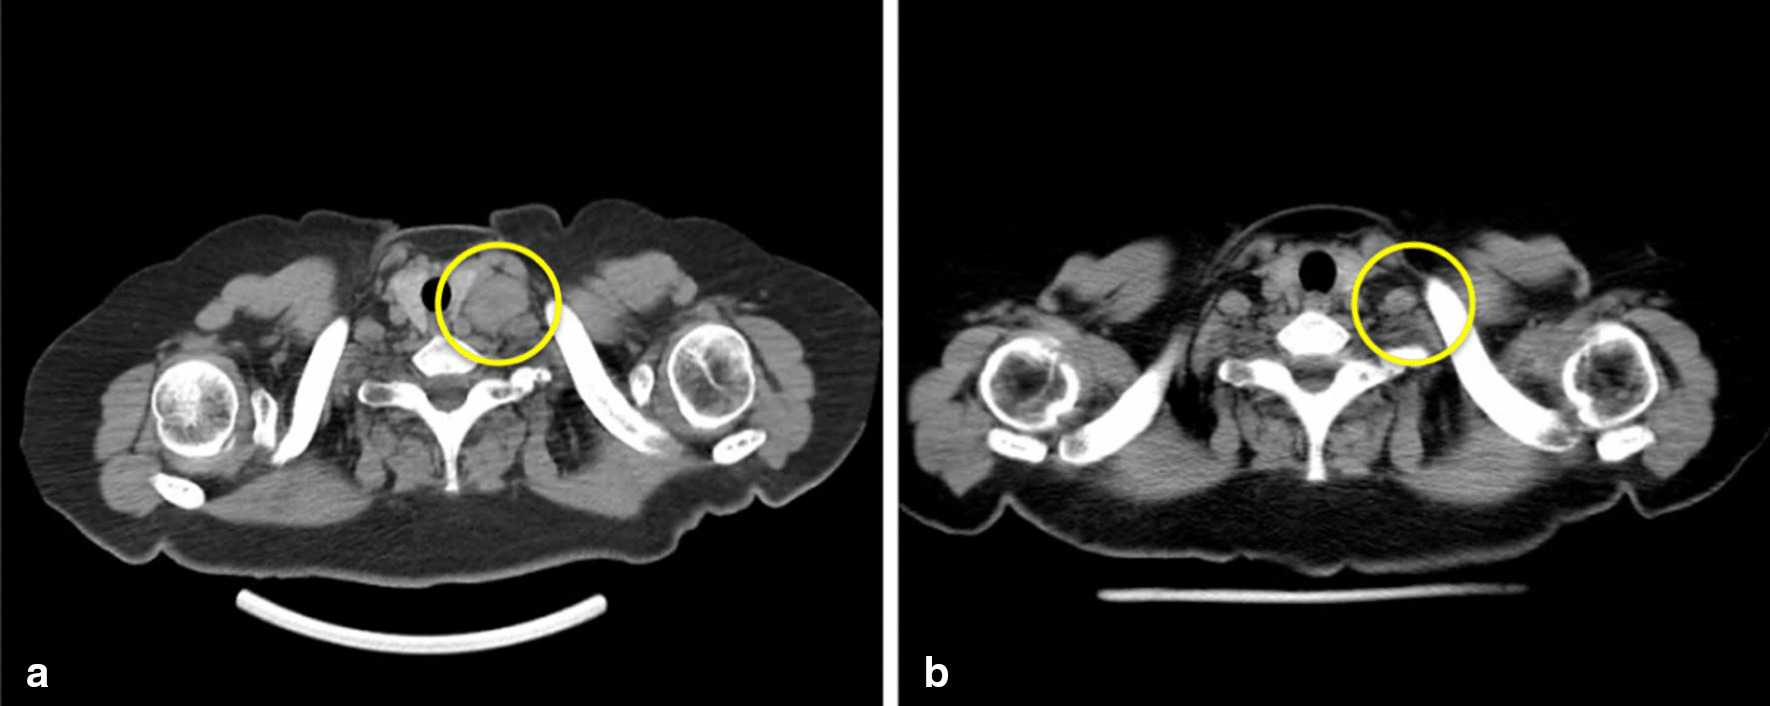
The contrast-enhanced CT showed the cervical lymph nodes on admission (b) significantly shrank compared with 3 months ago (a)
